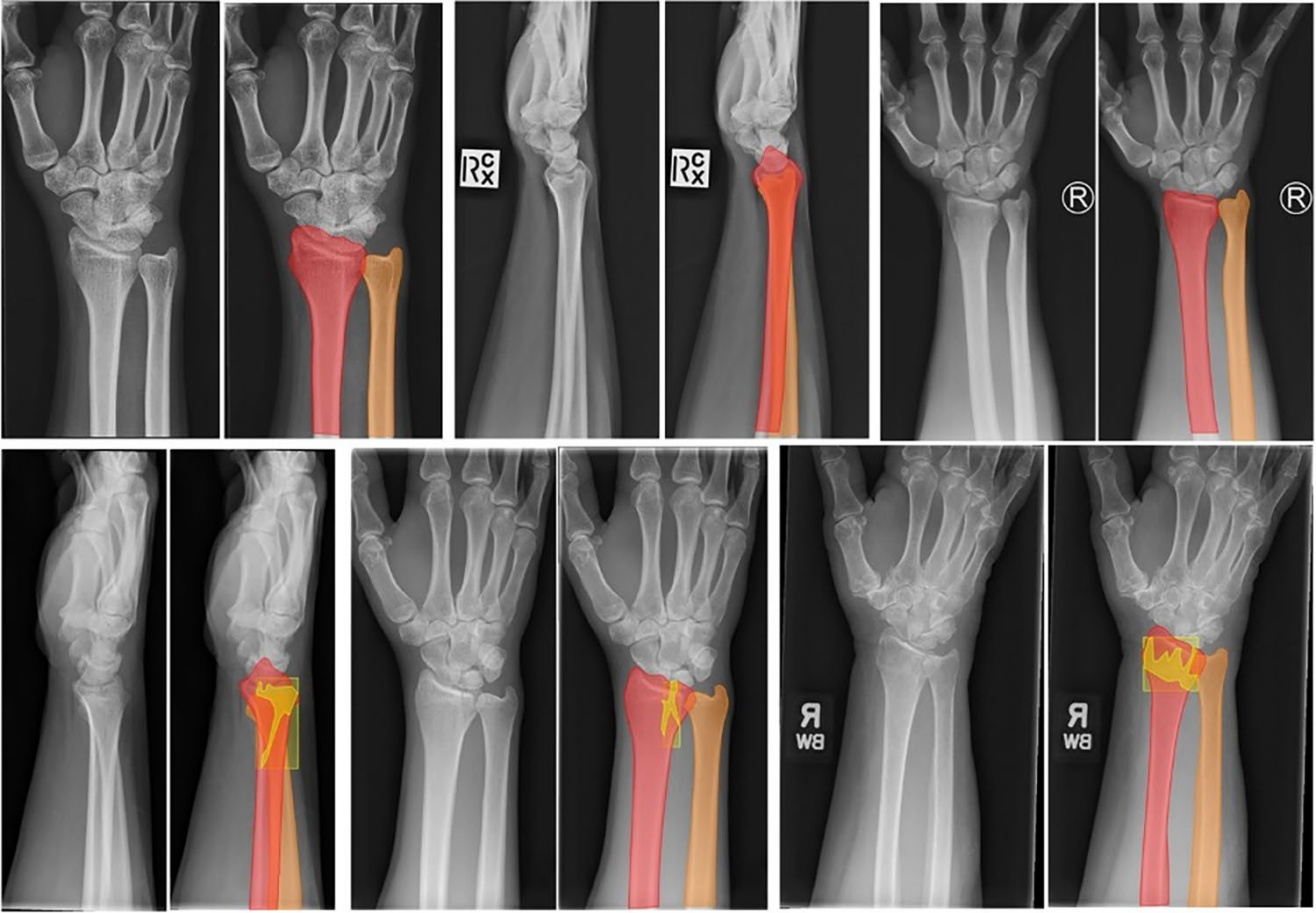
The upper row of images demonstrates, from left to right, the annotations of ulna and radius in non-fracture radiographs in oblique, lateral and PA view. The lower row of images shows the annotations of ulna, radius, and the region of interest (e.g., fracture site) using both a rectangular and polygon tool
